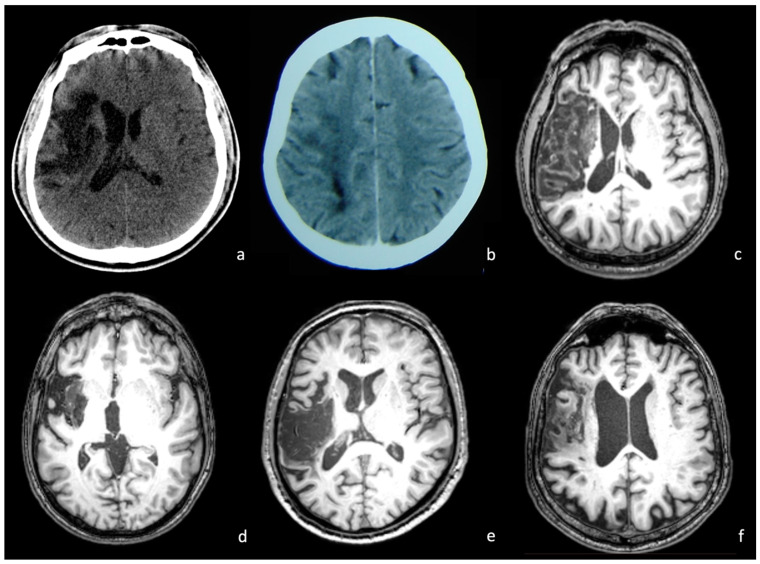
Examples of CT (a,b) and MRI (T1w) (c–f) axial images of stroke patients, based on which the neuroradiologists identified lesion locus. Each scan corresponds to a different patient.
